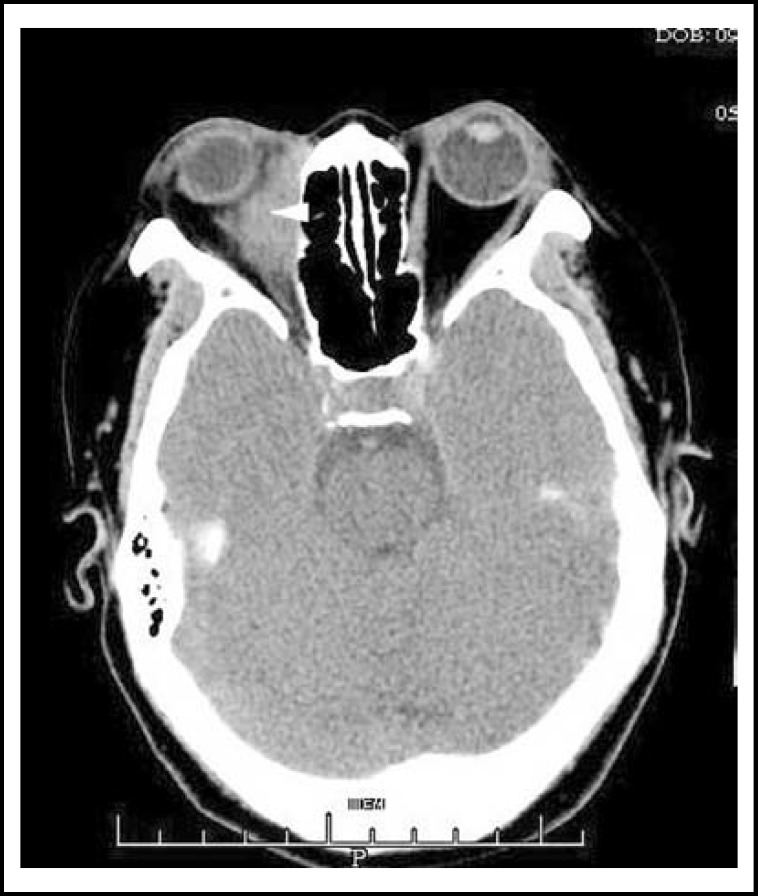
CT image showing a homogenous mass in right orbit (Arrow
